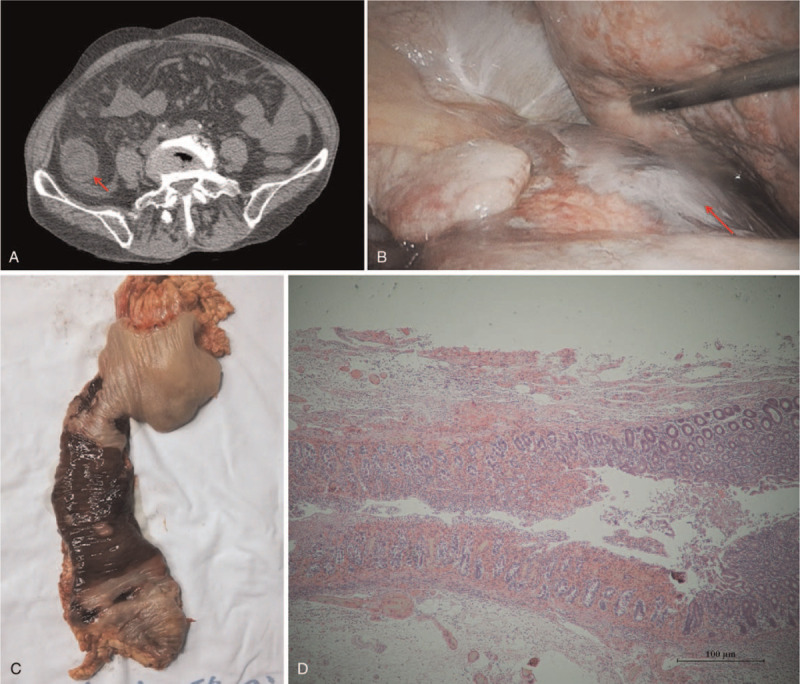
A: Abdominal CT showing swelling and thickening of the right colon and peritoneal effusion (arrow). B, C: Observation of necrosis in the hepatic flexure of the colon (arrow). D: Pathologic examination revealing large necrotic areas of colonic epithelium and abundant neutrophil infiltrations with abscess formation.
